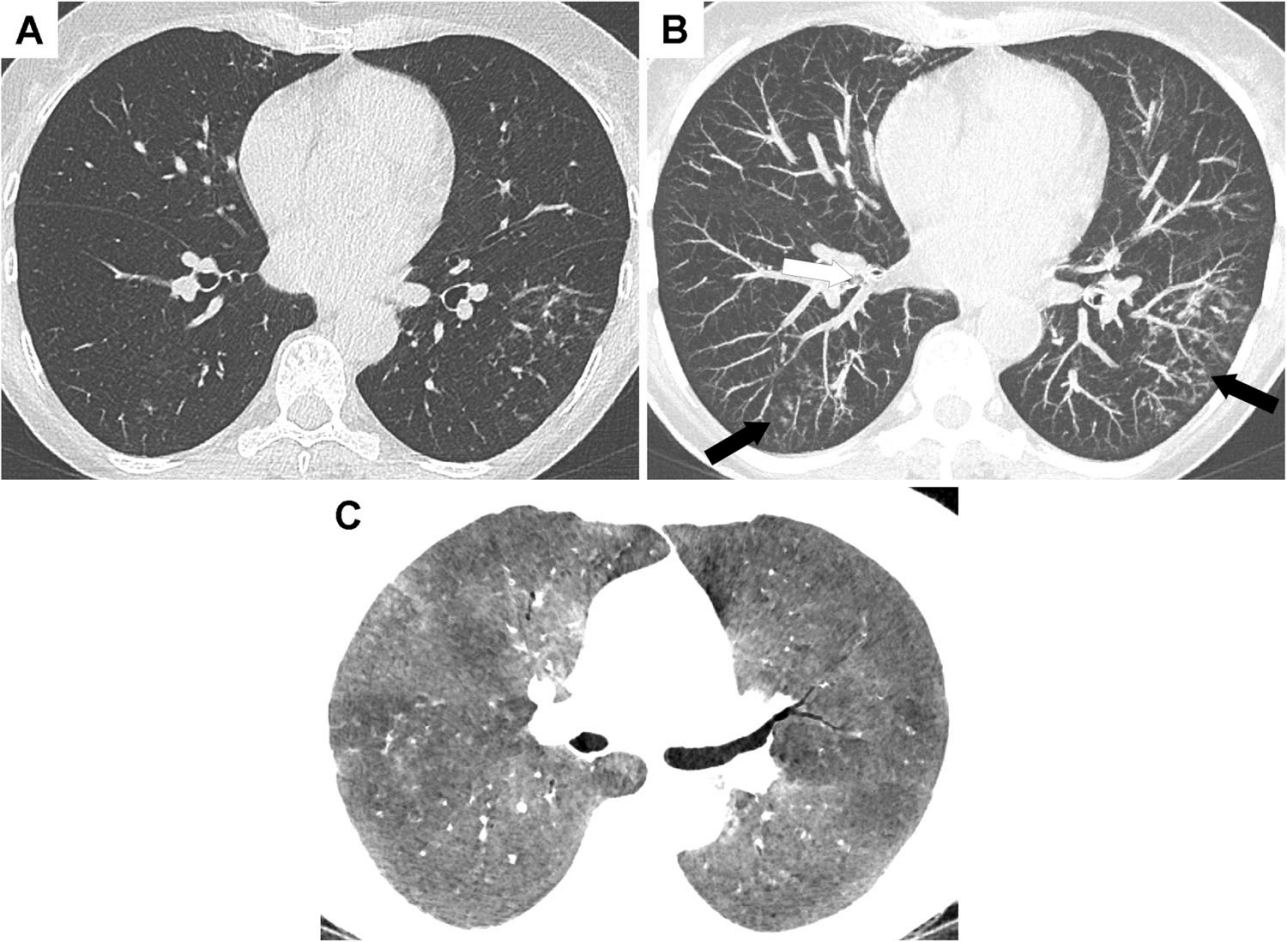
Small airways disease in a 60-year-old patient with ulcerative colitis. A Axial CT images show centrilobular nodules predominating in the right lower lobe. B Maximum intensity projection helps detect bilateral centrilobular nodules (arrows), which have a tree-in-bud appearance. C Minimum intensity projection shows the heterogeneity in the lung attenuation, which is related to diffuse mosaic perfusion pattern
